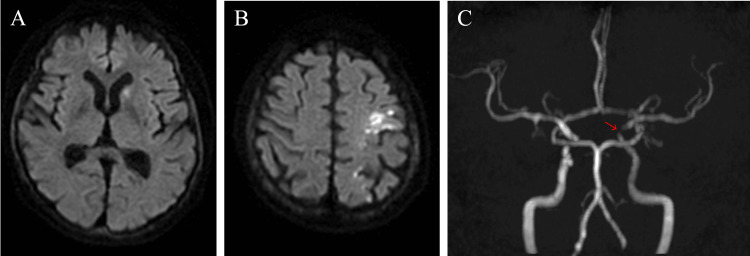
(A and B) Brain magnetic resonance imaging and (C) angiography at admission. Hyperintense lesions in the territory of the anterior branch of the left middle cerebral artery on axial diffusion-weighted imaging (A and B) and severe stenosis of the left internal carotid artery (C, arrow).
